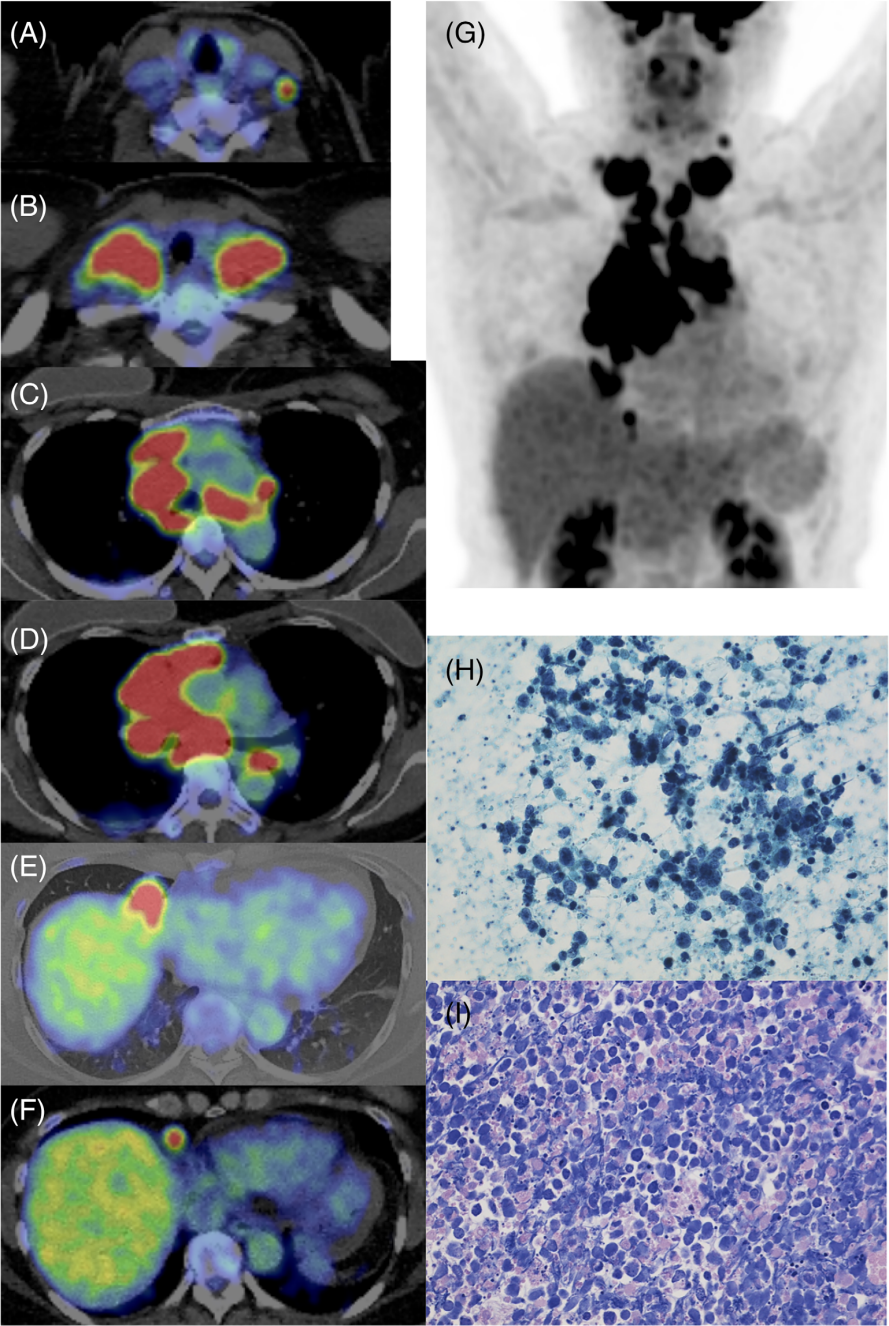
A 50‐year‐old woman underwent positron emission tomography‐computed tomography (PET‐CT) scan and aspiration cytology examination and was diagnosed with intrathoracic extensive‐stage small cell lung cancer (SCLC). Axial fused PET‐CT images (A–F) and anterior view of the maximum intensity projection (MIP) image of the PET‐CT scan (G) revealed increased [18F] fluorodeoxyglucose uptake of the bilateral supraclavicular, bilateral mediastinal and right pericardiac lymph nodes (LNs) and the right middle lobe tumour and a small amount of right pleural effusion. Aspiration cytology (H) and cell block preparation (I) of the left supraclavicular LN demonstrated SCLC
